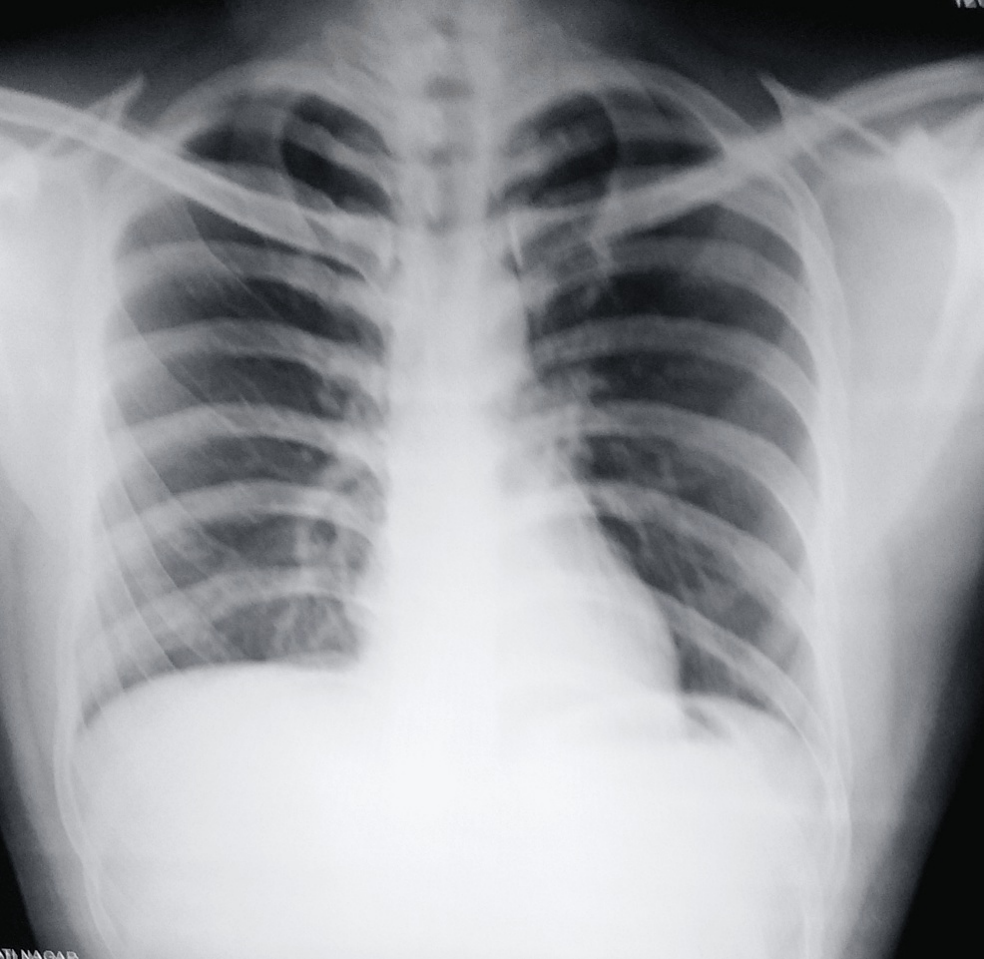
A plain chest radiograph (P-A) view P-A: Posteroanterior
